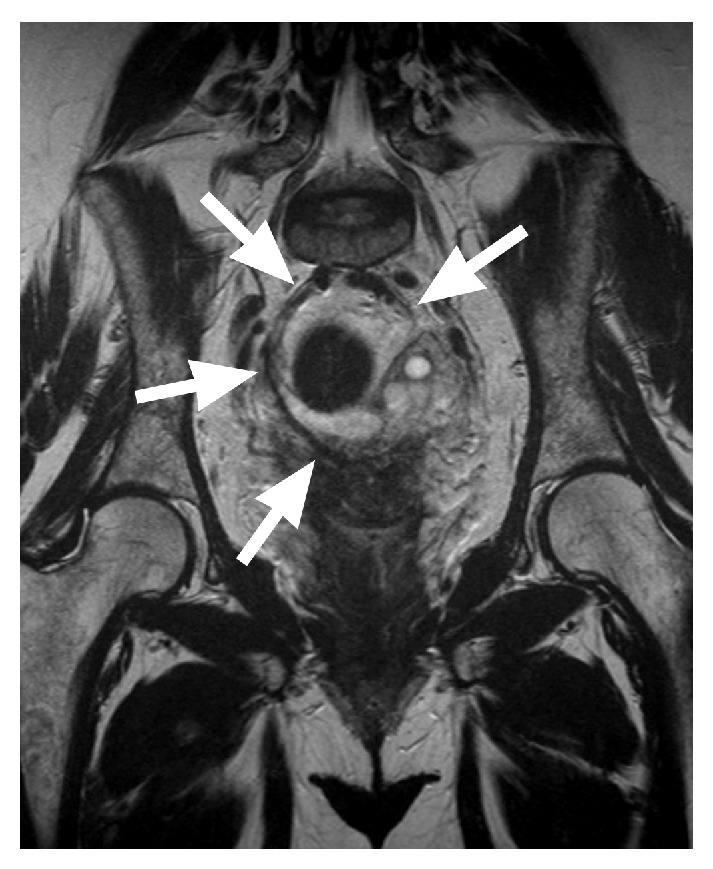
Coronal subvolume minimum intensity projection of a T2-weighted dataset, displaying the implant with a low signal intensity (arrows), comparable to the signal of muscle tissue.
